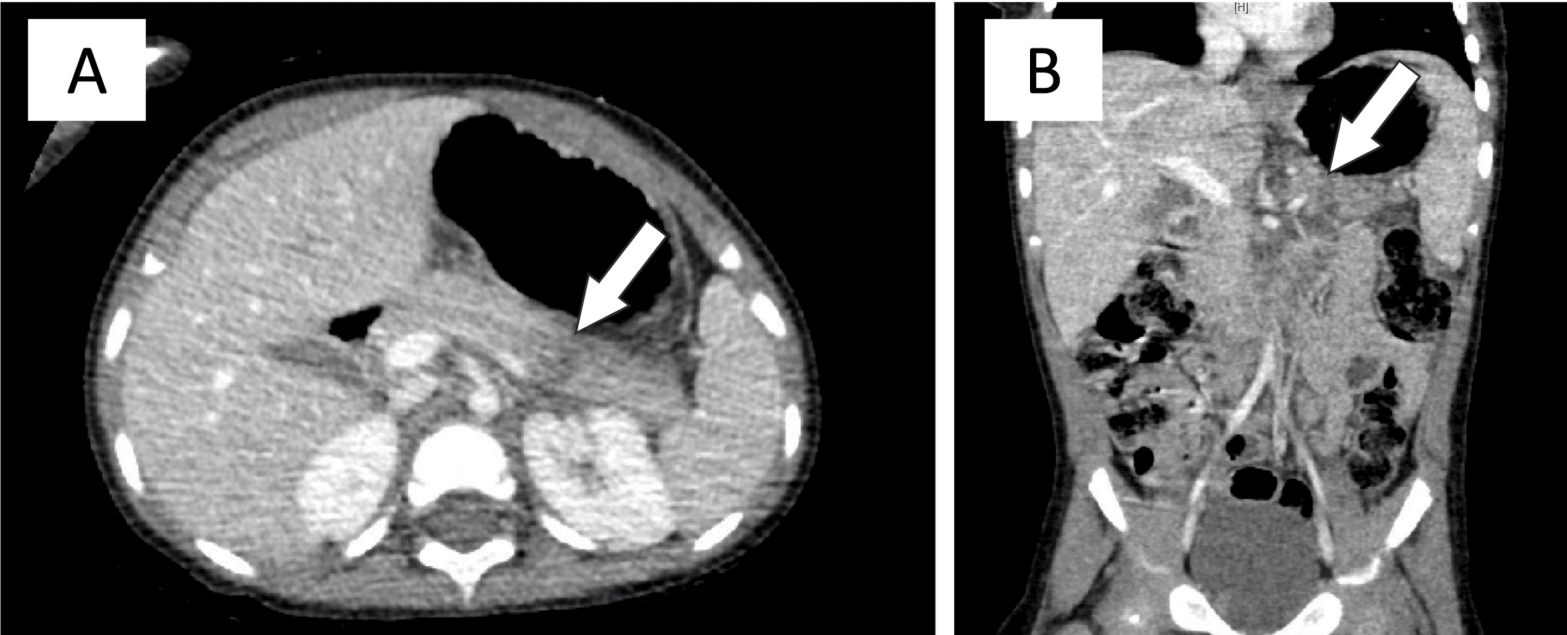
Case 1: Abdominal CT with contrast. (A) Axial view. Transverse lucency (white arrow) through the distal body and tail of the pancreas with adjacent edema in the pararenal space, extending toward the inferior spleen. A grade III left kidney injury is also observed. (B) Coronal view. Transverse lucency (white arrow) can be observed. Findings were compatible with a grade III pancreatic injury.
